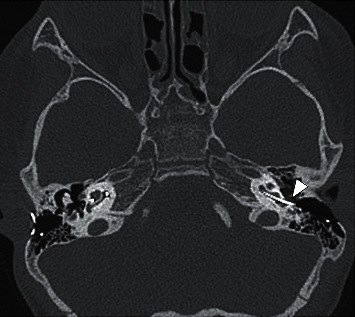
Computed tomography scan of the temporal bone with the arrowhead pointing to the electrodes outside the cochlear.
